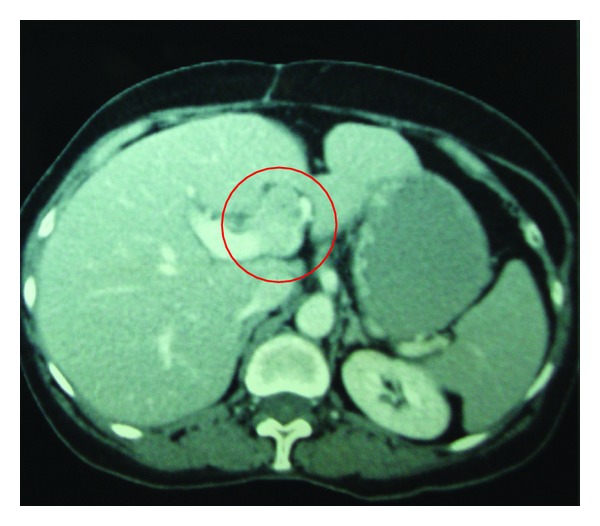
CT scan showing mass lesion in segment 4 abutting the left branch of portal vein.
